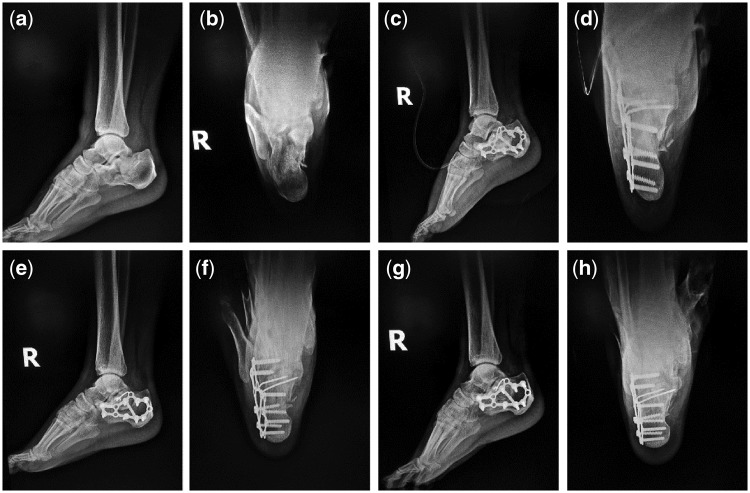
Plain radiographs of the third typical case. (a and b) preoperative lateral and anteroposterior films; (c and d) immediately postoperative lateral and anteroposterior films; (e and f) 3 months postoperative lateral and anteroposterior films; (g and h) 12 months postoperative lateral and anteroposterior films)
